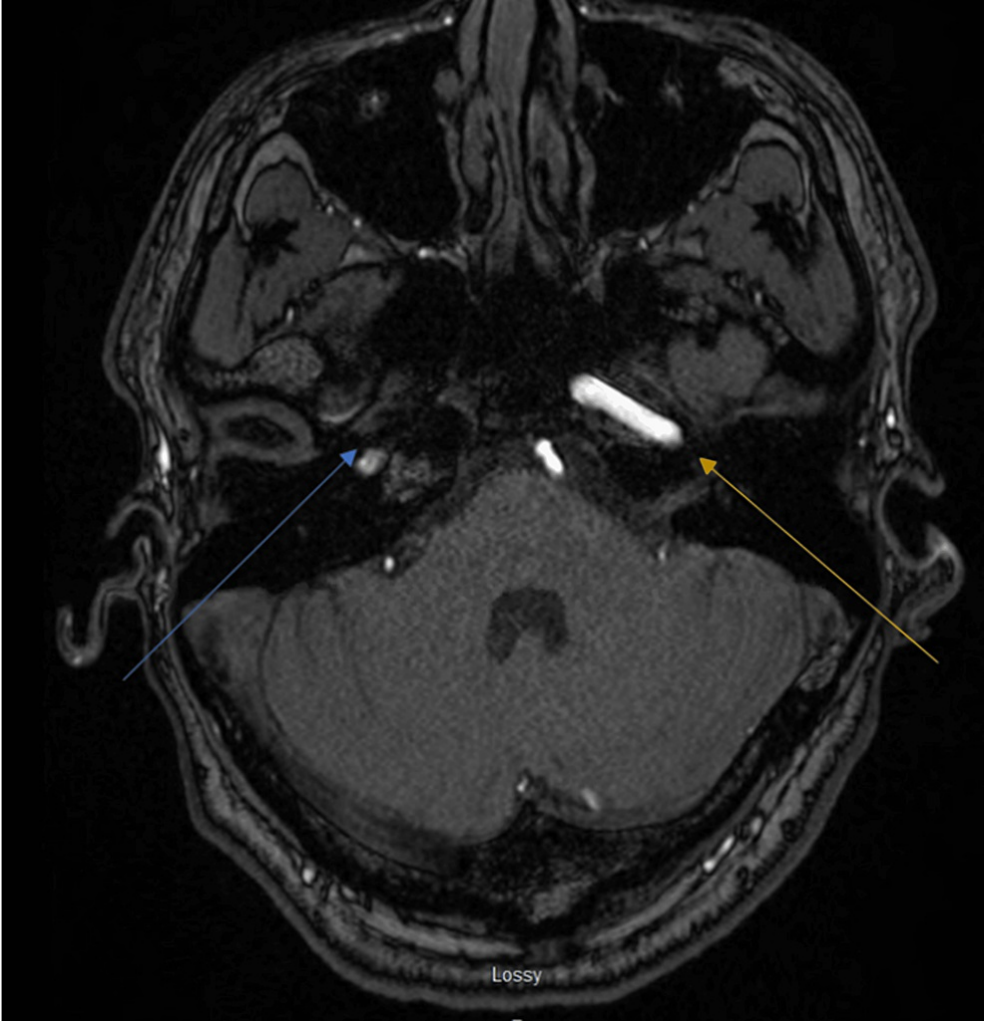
Magnetic resonance imaging of the brain. The blue arrows show very diminutive flow in the right internal carotid artery just above its origin. Diminutive signal but some flow in the right petrous internal carotid artery and distal internal carotid artery can be seen, suspected with retrograde ophthalmic artery. Some flow can also be noted in the right M1 and M2 middle cerebral arteries without any obvious large vessel clots.
